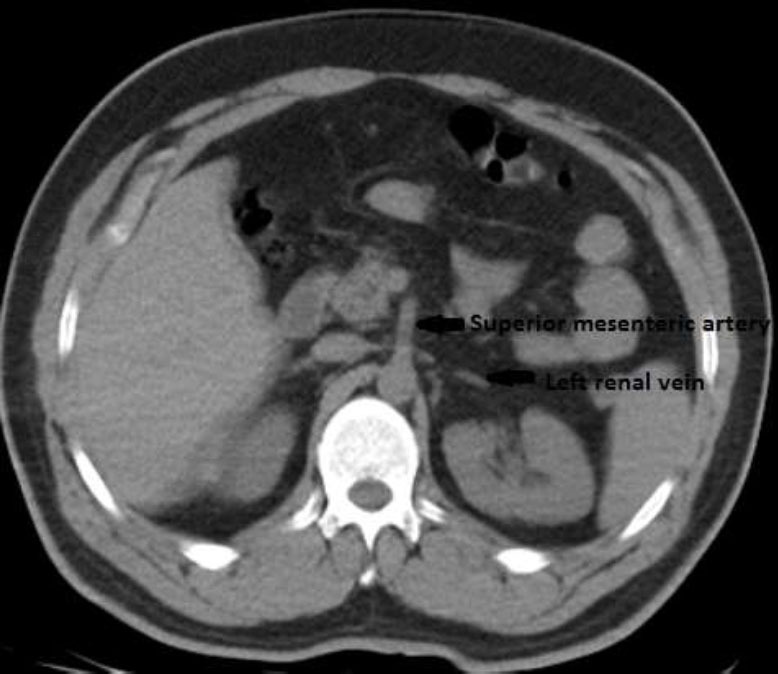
Nutcracker renal vein syndrome in abdomen CT.
